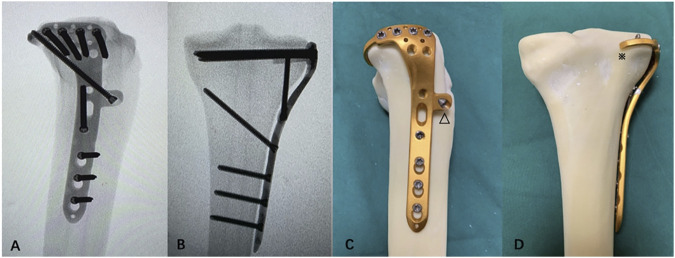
Mock-ups of the novel plate in intact synbones. (A) Lateral view of the X-ray image. (B) Anteroposterior view of the X-ray image. (C) Lateral view of the novel plate. (D) Anteroposterior view of the novel plate. The (△) anterior wing and (※) “hoop hook.”
